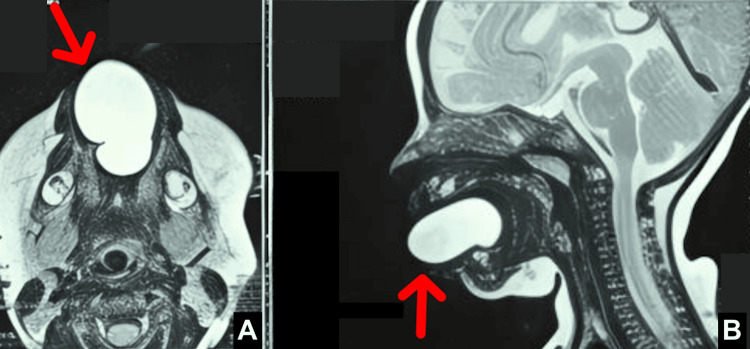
MRI showing a well-circumscribed, non-enhancing T1-hypointense/T2-hyperintense cystic lesion arising from the ventral surface of the tongue along its middle and anterior portion Magnetic resonance imaging of the oral cavity demonstrates a well-circumscribed, biloculated cystic lesion arising from the ventral aspect of the anterior tongue. (A) Axial T2-weighted image shows a markedly hyperintense biloculated lesion occupying the anterior oral cavity. (B) Sagittal T2-weighted image demonstrates anterior extension of the lesion beyond the labial folds, with significant mass effect on the dorsal surface and base of the tongue, resulting in posterosuperior displacement.
